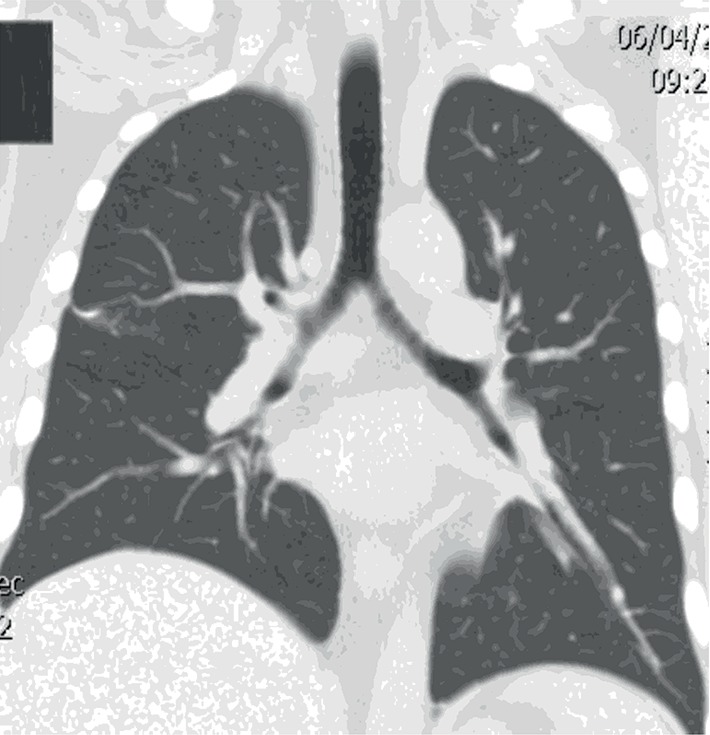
Coronal CT chest at lung window showing complete resolution of infiltrates, two months post discharge from the hospital.
